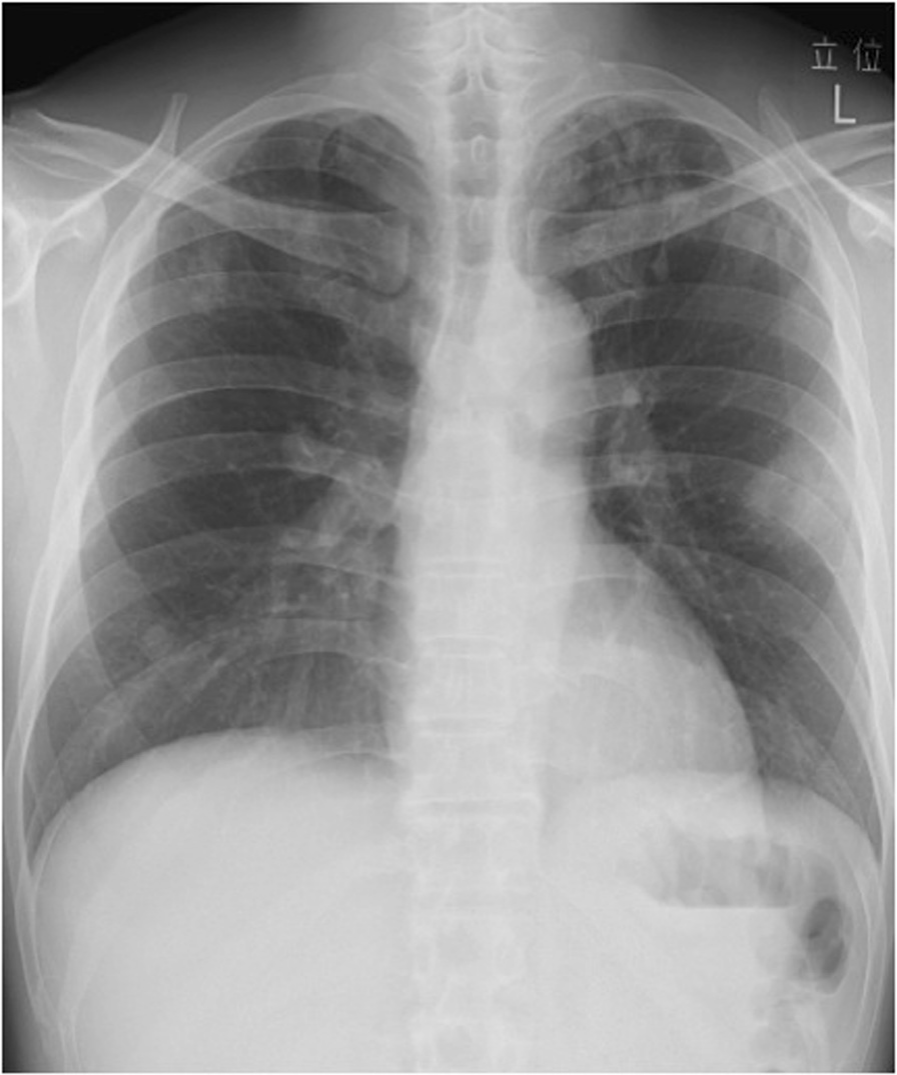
Chest X-ray in June 2006 showed a new solid nodule in the left lung field that gradually enlarged.
